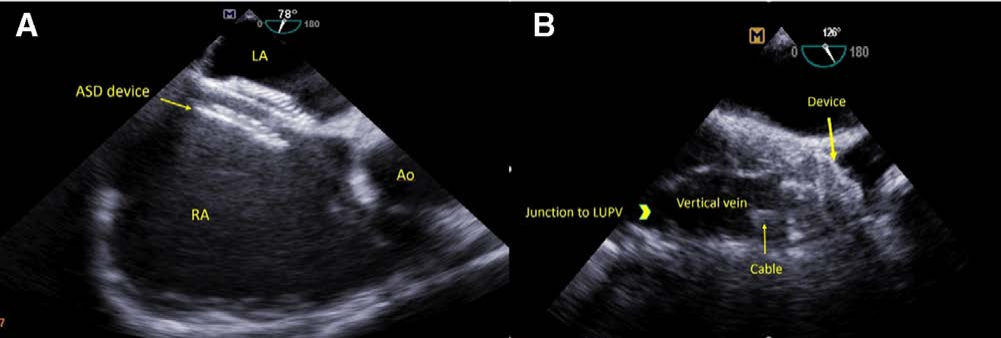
Transesophageal echocardiography images. The images show (a) the ASD device deployed in an appropriate position and (b) the VSD occluder device with complete occlusion of the vertical vein. Ao: aorta, ASD = atrial septal defect, LA = left atrium, LUPV = left upper pulmonary vein, RA = right atrium, VSD = ventricular septal defect.
